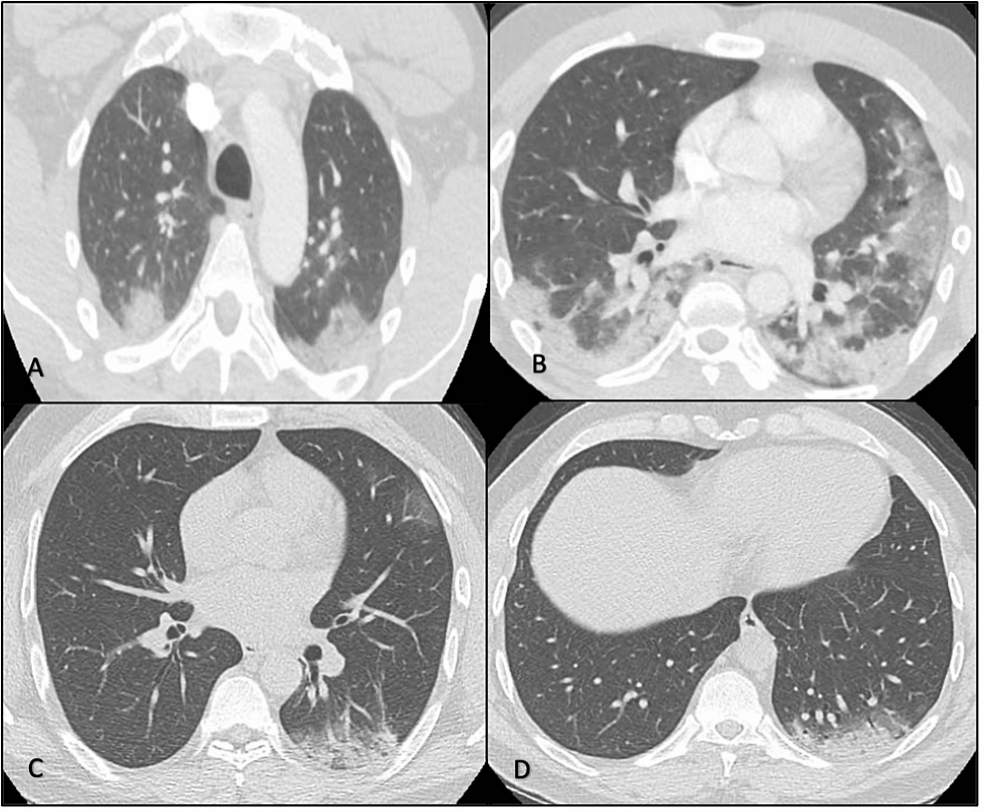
A and B: CT scan of the chest with contrast showing patchy and consolidative airspace opacities involving bilateral upper and lower lobes, and additional nonspecific ground glass in bilateral lower lobes. No pulmonary embolism was noticed. C and D: Interval resolution of prior findings with residual consolidation in the posterior left lower lobe.
